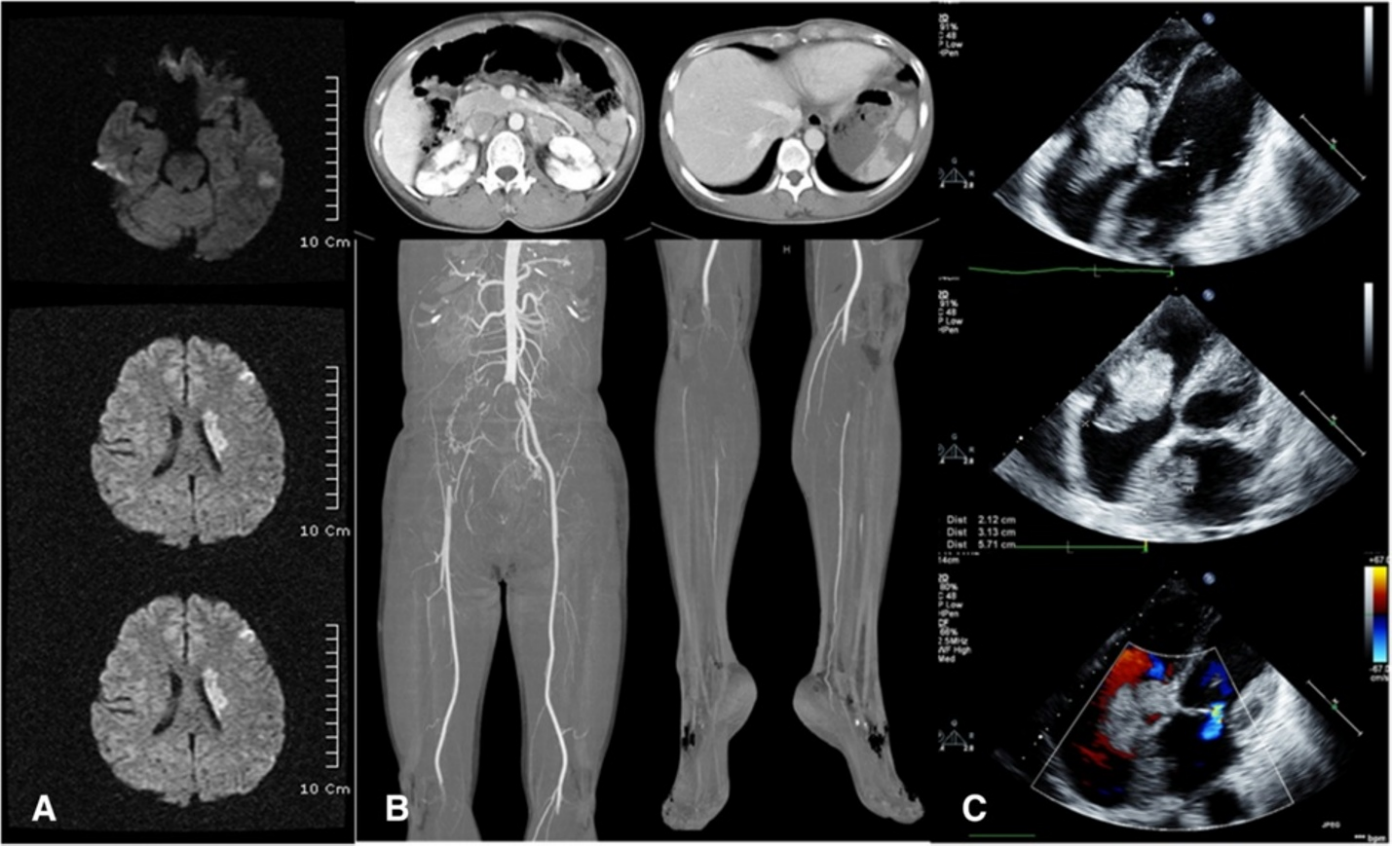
Brain MRI with diffusion, CTA and transthoracic echocardiography. (A) Acute multifocal territorial infarct, frontotemporoparietal lobe area and striatocapsule with minimal swelling in the left mid-cerebral artery. (B) Multifocal spleen and renal infarctions in bilateral kidneys and complete segmental obstruction of the aortic bifurcation, bilateral common iliac arteries, right internal iliac artery and bilateral popliteal arteries. (C) A large (5.7×3 cm) round and pedunculated homogeneous mass that occupied most of the RA and prolapsed through the tricuspid valve with functional tricuspid stenosis, and another large (3.8×2 cm) villous mass that was attached to the septal side with no stalk in the large LA with mild MR.
